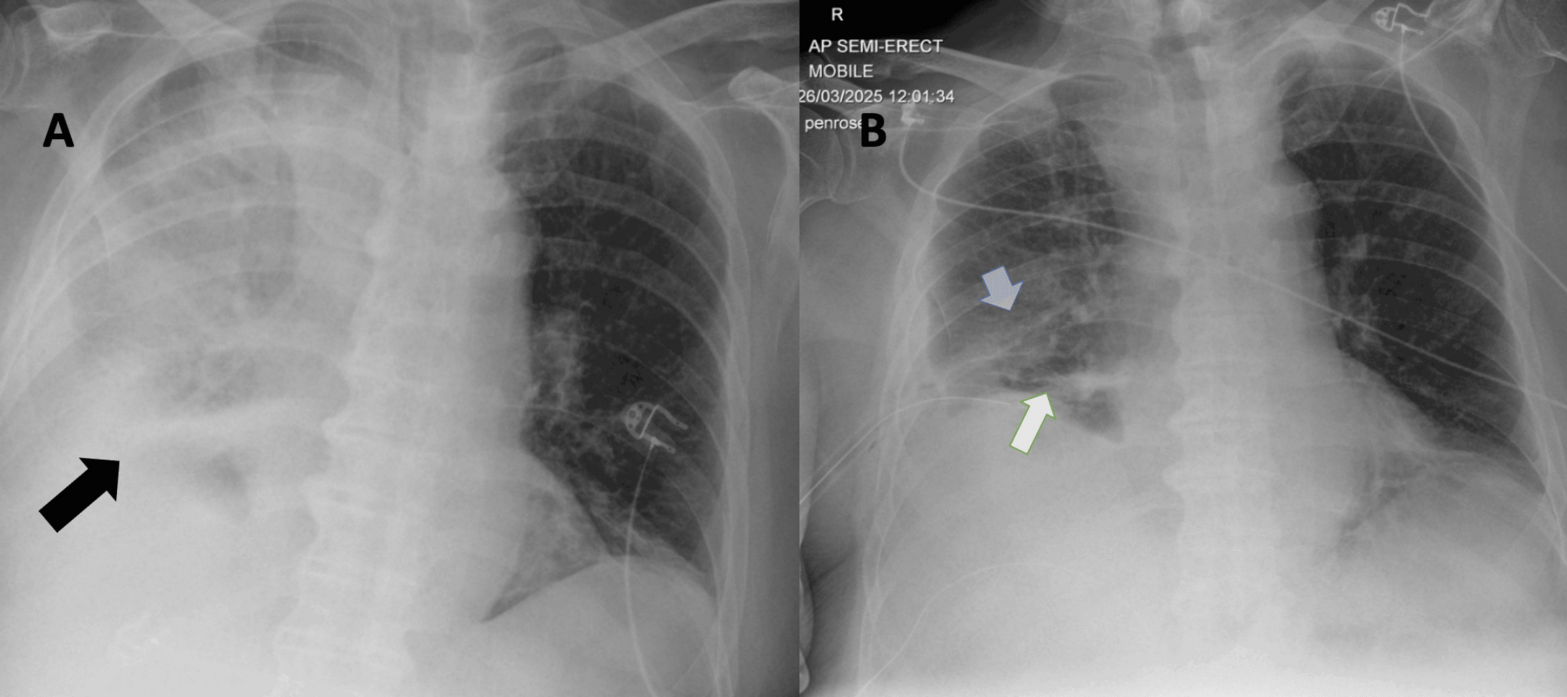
Serial chest radiographs (anteroposterior, semi-erect) (A) 25^th^ March, 2025: Extensive right-sided opacification consistent with consolidation and associated pleural effusion (black arrow); (B) 26^th^ March, 2025: Post chest-drain insertion with one drain tip projected over the right mid-zone (blue arrow) and another within the cardiophrenic recess (white arrow); interval improvement in right-sided aeration.
